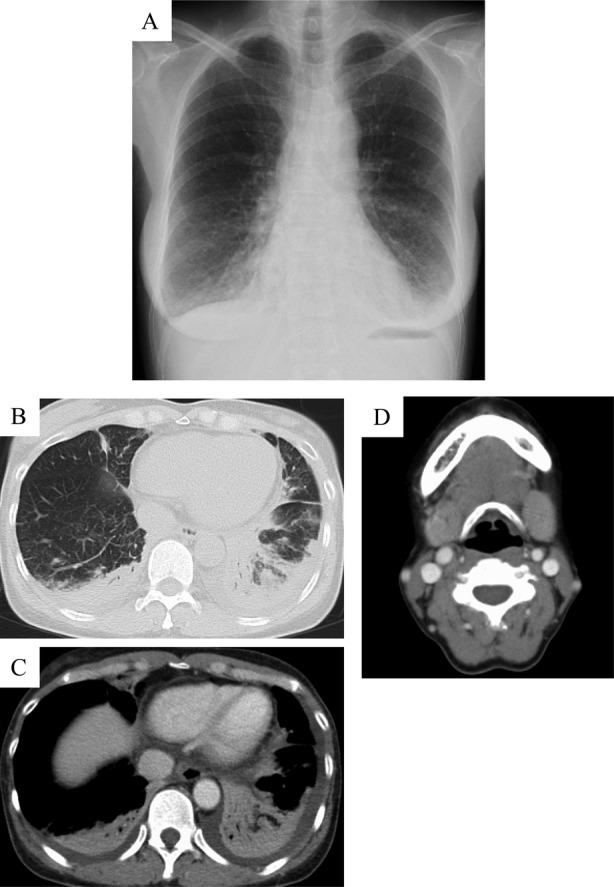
Chest radiography and computed tomography (CT) findings in a 51‐year‐old woman with eosinophilic granulomatous polyangiitis (EGPA). (A) Chest radiography shows infiltrates in the bilateral lower lung fields. (B, C) Chest CT shows dense infiltrations in the bilateral lower lobe and bilateral pleural effusion. (D) Head CT shows marked swelling of the bilateral submandibular glands.
